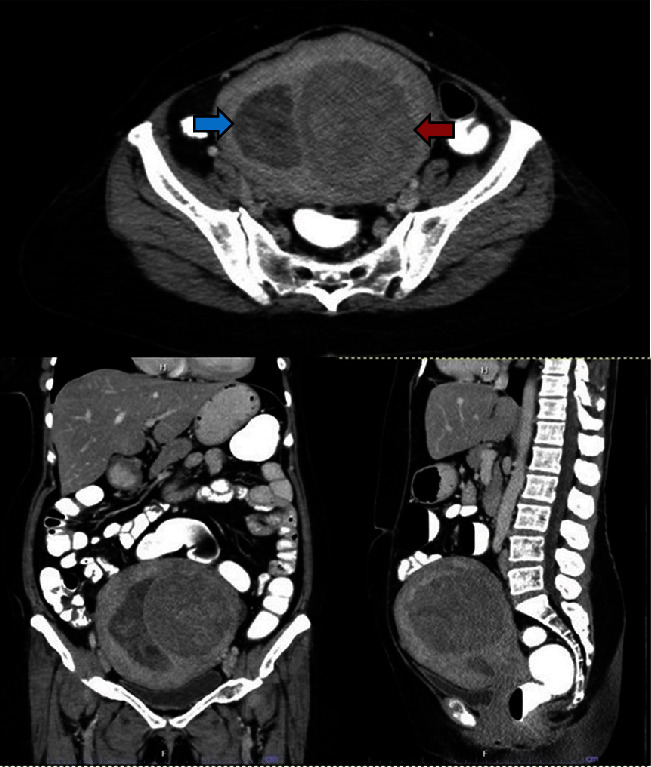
Abdominal CT scan demonstrated enlarged uterus with well-defined heterogenous enhancing lesions at the left side of uterine wall (red arrow). Heterogenous hypodense lesion in the right side of the uterine cavity with 3.8 cm thickness (blue arrow).
